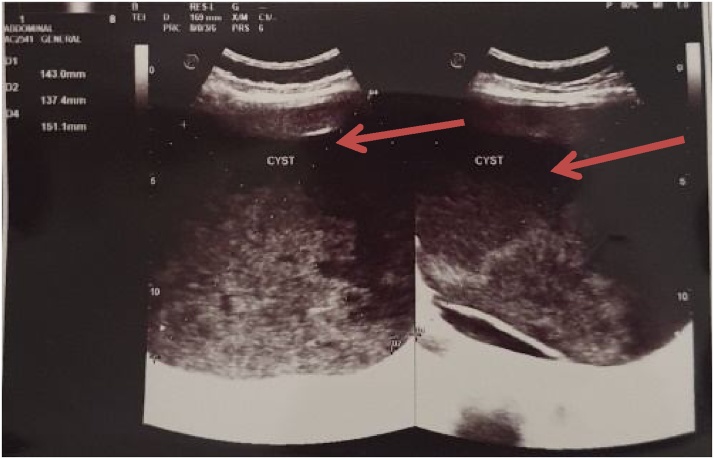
Pancreatic head and liver cyst were revealed during abdominal ultrasonography.
